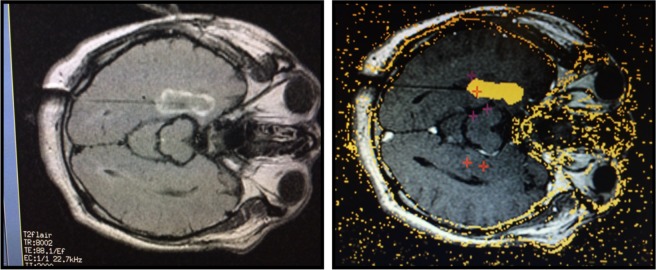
Real time T2-FLAIR (left) and thermal (right) MRI brain images of immediate post-operative brain demonstrating blood brain barrier disruption outline and of permanent brain tissue destruction during stereotactic laser amygdalohippocampotomy (SLAH) (orange).
